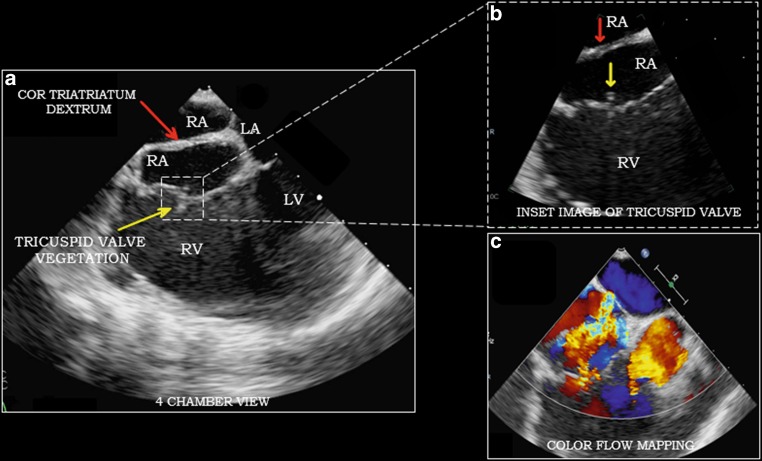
a, b 2D transoesophageal echocardiography, apical mid-oesophageal four-chamber view of the heart showing presence of a membrane separating the right atrium into two parts (*red arrow*) and vegetation over tricuspid valve (*yellow arrow*); c Colour flow Doppler study showing regurgitation at tricuspid valve and back flow of blood through the incomplete septum of cor triatriatum dexter. (*LA* left atrium,* RA* right atrium,* RV* right ventricle,* LV* left ventricle)
